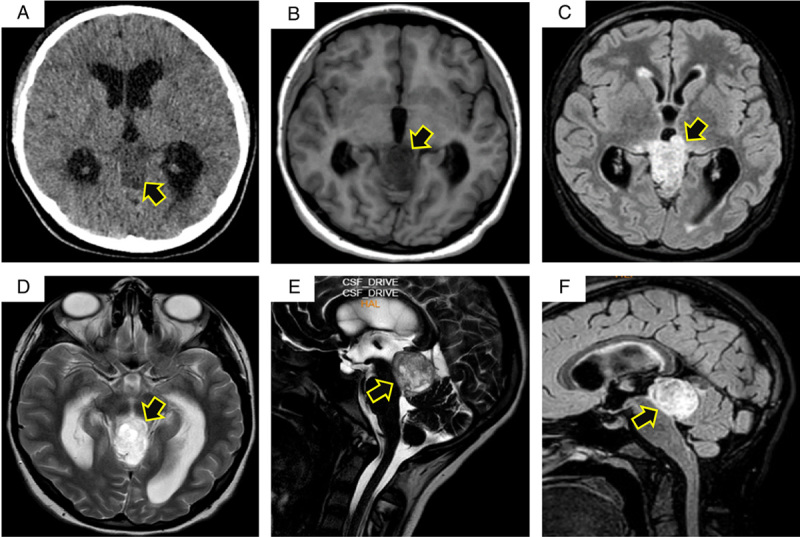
(A) Axial nonenhanced computed tomography scan showing a hypodense mass; (B) T1 sequence axial MRI; (C) FLAIR (fluid-attenuated inversion recovery) sequence axial MRI; (D) T2 sequence axial MRI; (E) FIESTA (fast imaging employing steady-state acquisition) sequence sagittal MRI; (F) FLAIR sequence sagittal MRI. CSF, cerebrospinal fluid.
